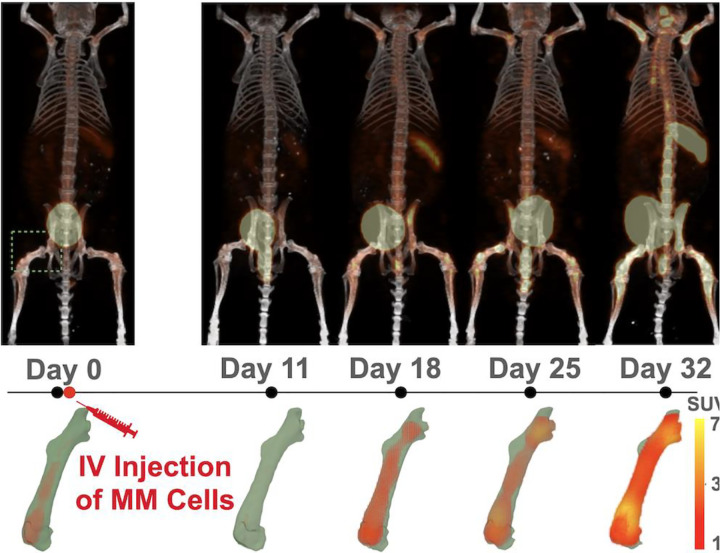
In a longitudinal ^64^Cu-LLP2A PET/CT study, we demonstrated that by accurately segmenting the bone, we can numerically trace how the overlapped SUV values change for each bone ROI over time
